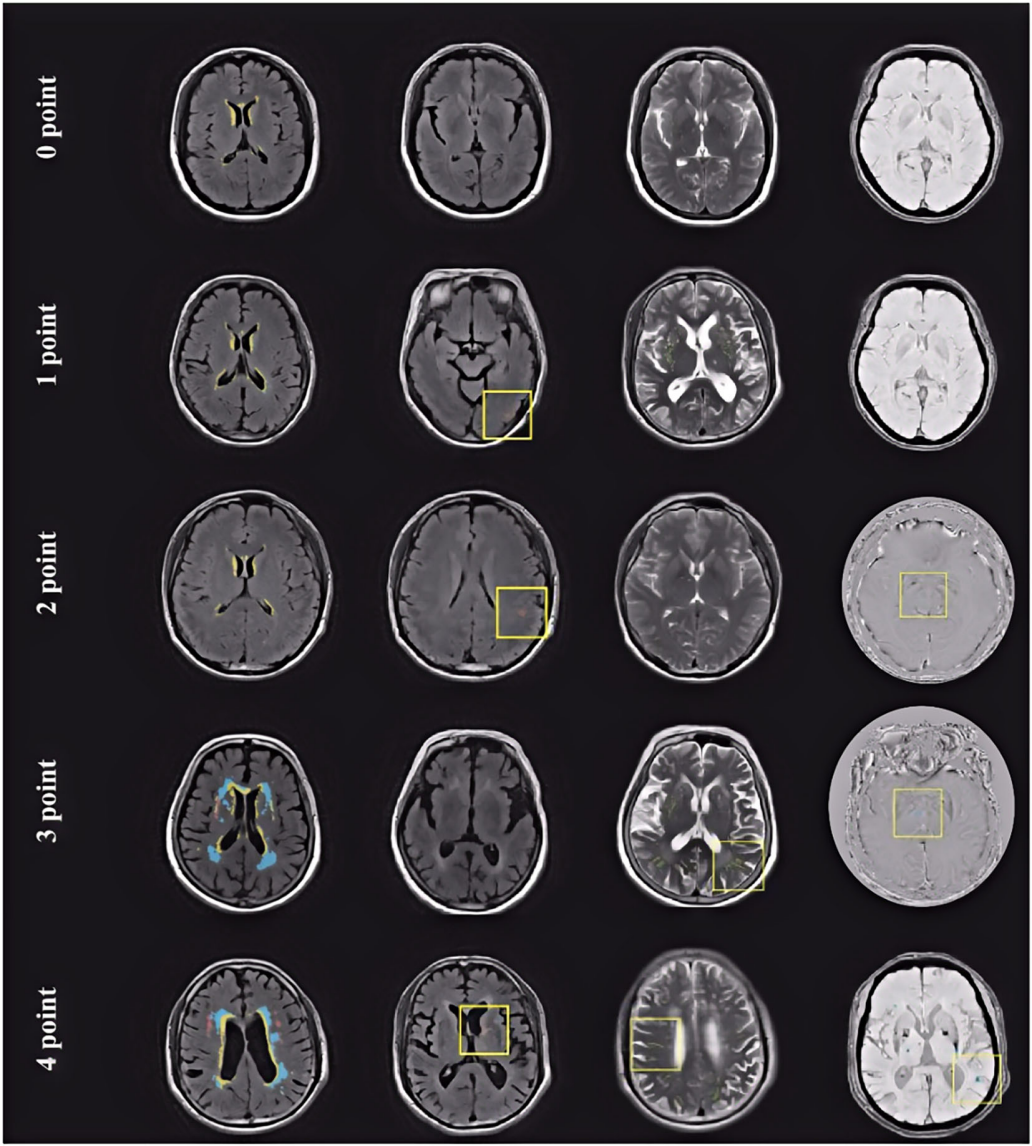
MRI images from patients with different cerebral small vessel disease scores.
